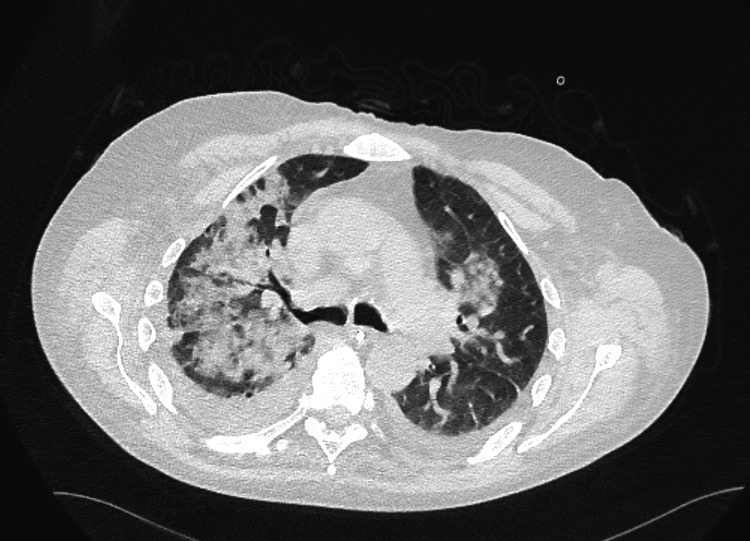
Computed tomography of the chest showing bilateral pulmonary haemorrhages, more pronounced in the right lung. Bilateral high-attenuation ground-glass and patchy consolidation, more pronounced on the right, suggest acute alveolar haemorrhage from a vasculitic process.
